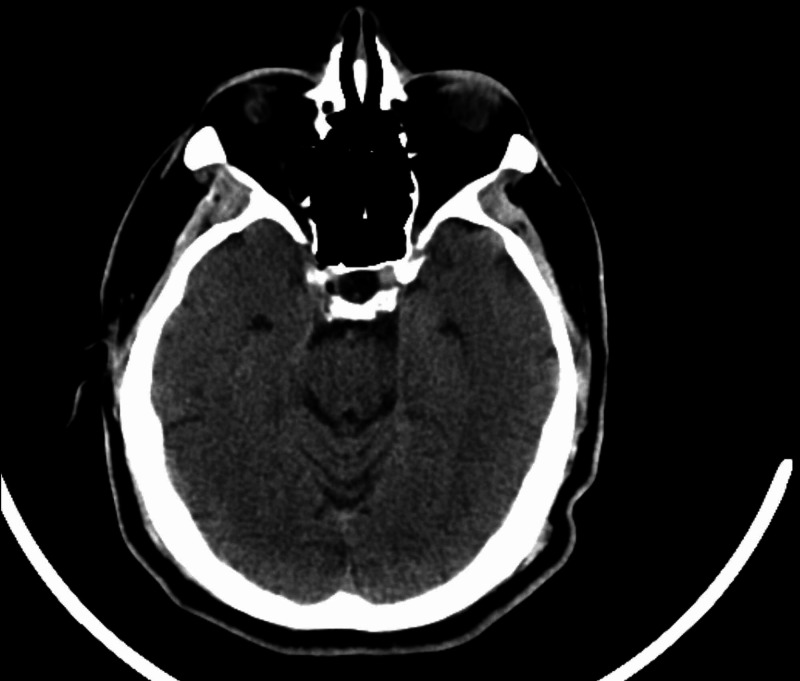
Computed tomography of the brain without contrast showed no signs of intracranial hemorrhage or brain infarction
